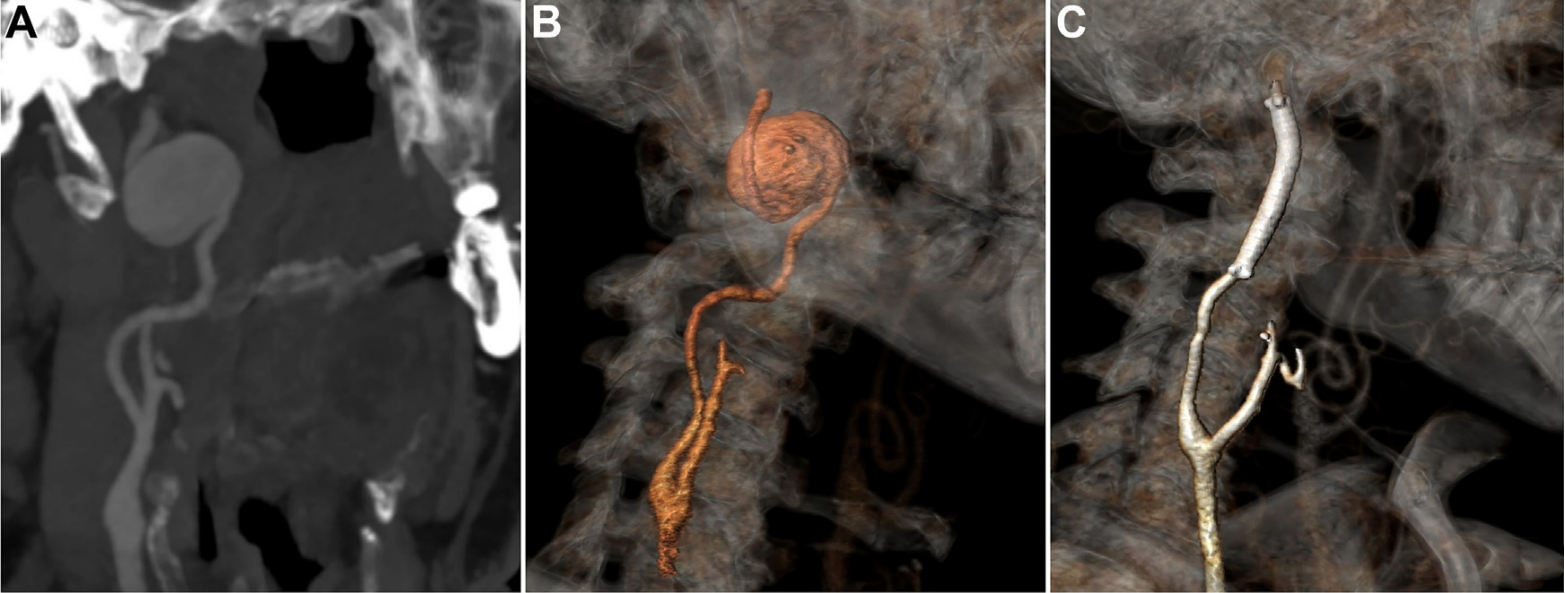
Preoperative computed tomography angiography (CTA) in a coronal view showing a distal saccular extracranial carotid artery aneurysm (CAA) Attigah classification Type I (A). Three-dimensional reconstructions of CTA at admission (B) and after Viabahn-assisted sutureless anastomosis (VASA) repair (C).
